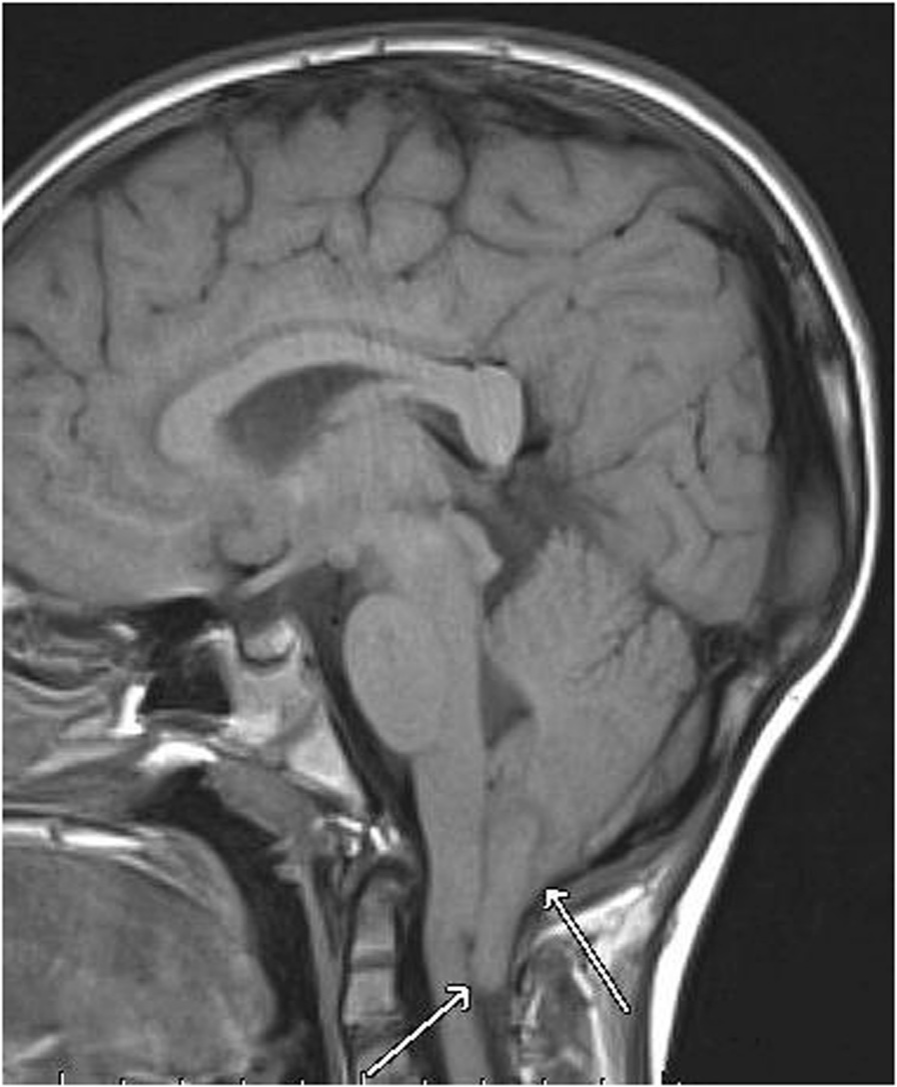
Cerebellar tonsils herniation on magnetic resonance imaging: Chiari malformation type I.
